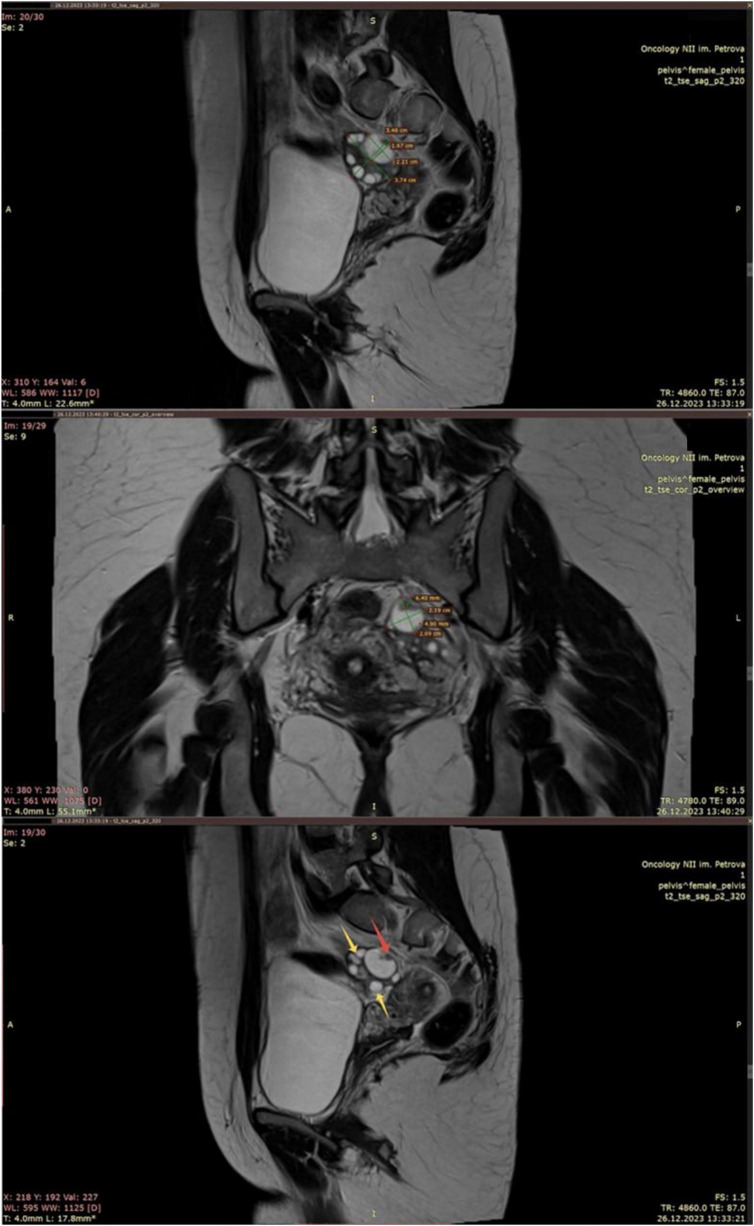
MRI image with intravenous contrast: recurrent tumor in the ovary with papillary growths along the internal capsule in two projections. MRI image with intravenous contrast: recurrent tumor in the ovary with papillary growths along the internal capsule in two projections. Red arrow shows recurrent tumor, yellow ones show antral follicles in the ovary.
